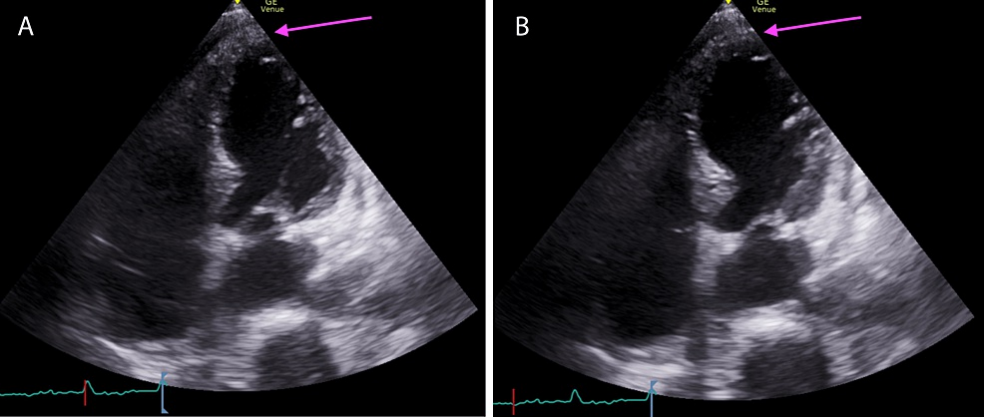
Echocardiogram Echocardiogram showed a decline in blood pressure after extubation on postoperative day 6. (A) Diastolic phase. (B) Systolic phase. The basal portion of the heart was contractile, but the apex was asystolic.
